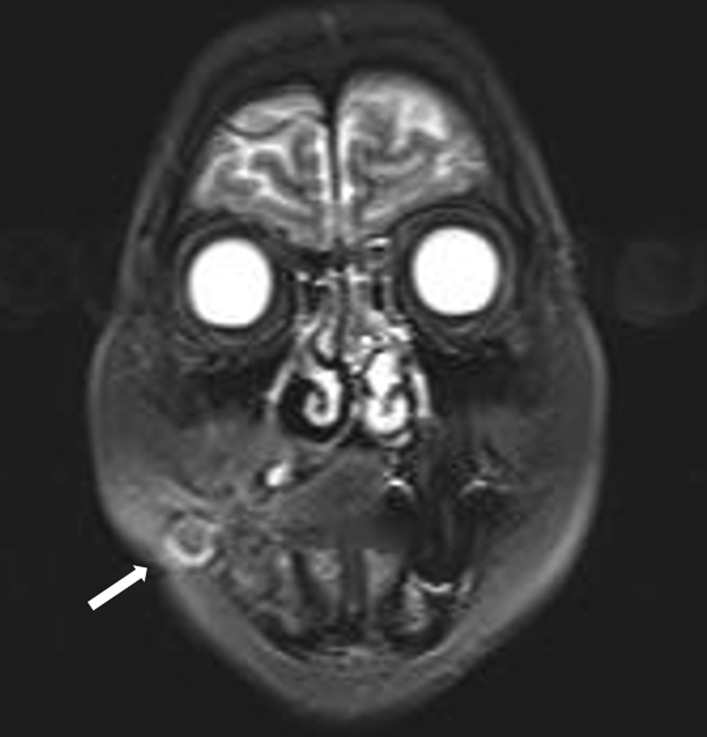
T2 fat-suppression magnetic resonance imaging demonstrating a mass in the right buccal region (arrow)
